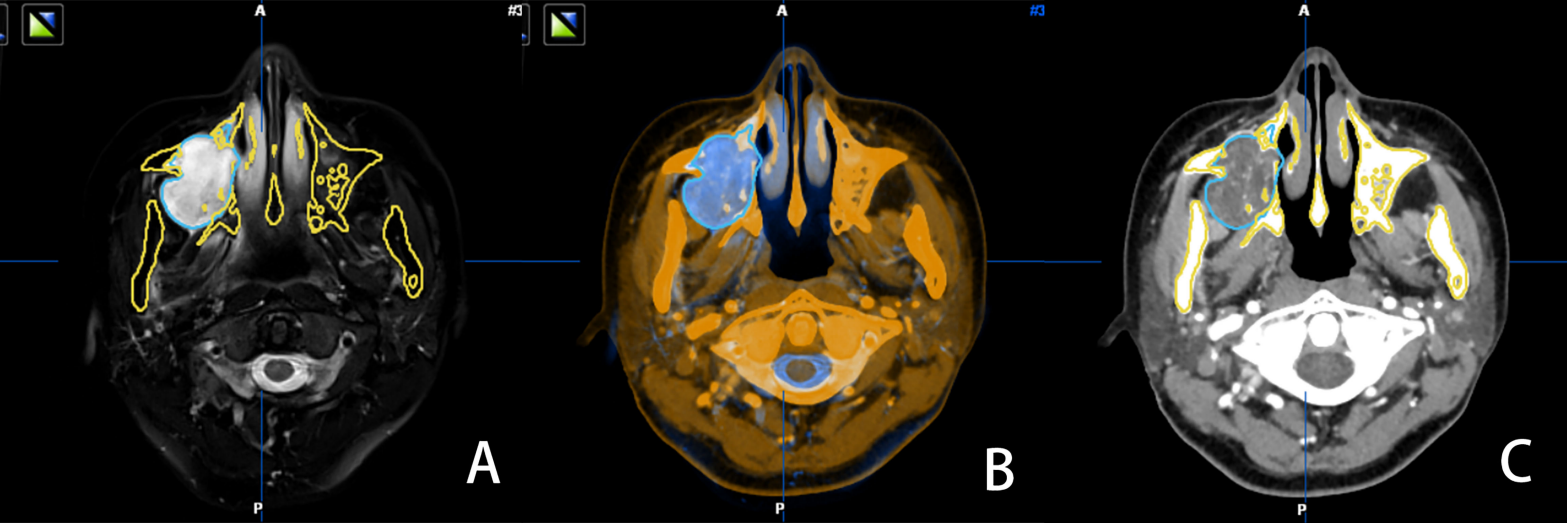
MRI image, with tumor boundary depicted in blue (A) the software automatically recognized the bone tissue structure of the CT and MRI images in the same slice and fused them (B) the tumor boundary is marked in blue in the CT image (C).
